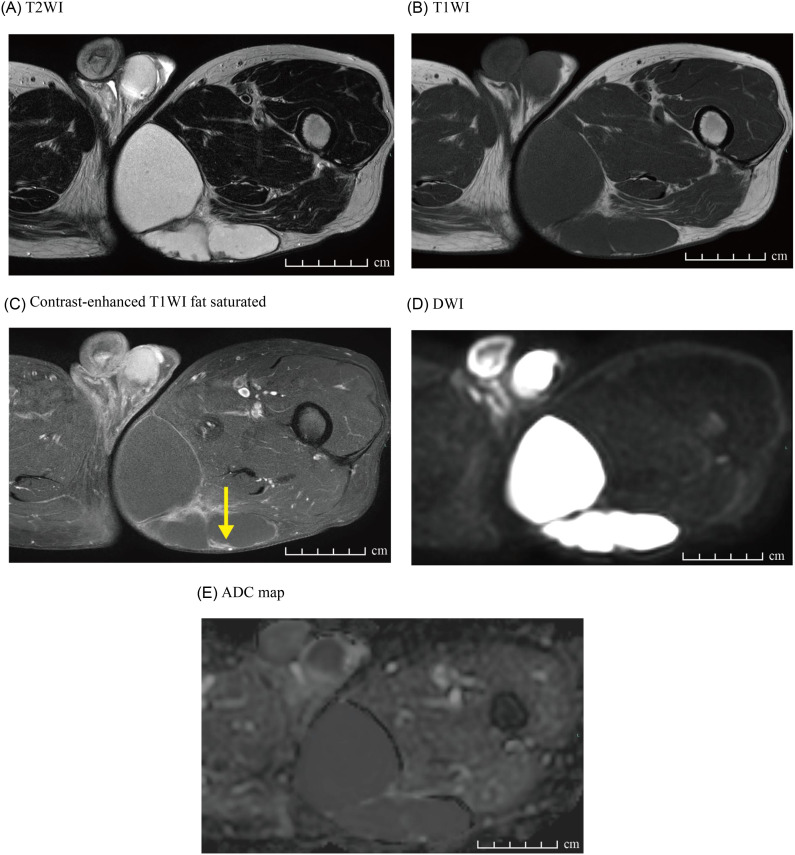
Case 1, a male in his 60s. Magnetic resonance imaging (MRI) is shown: (A) T2-weighted image (T2WI), (B) T1-weighted image (T1WI), (C) contrast-enhanced T1WI fat-saturated (a nodule is indicated by yellow arrow), (D) diffusion-weighted image (DWI), and (E) apparent diffusion coefficient (ADC) map.
